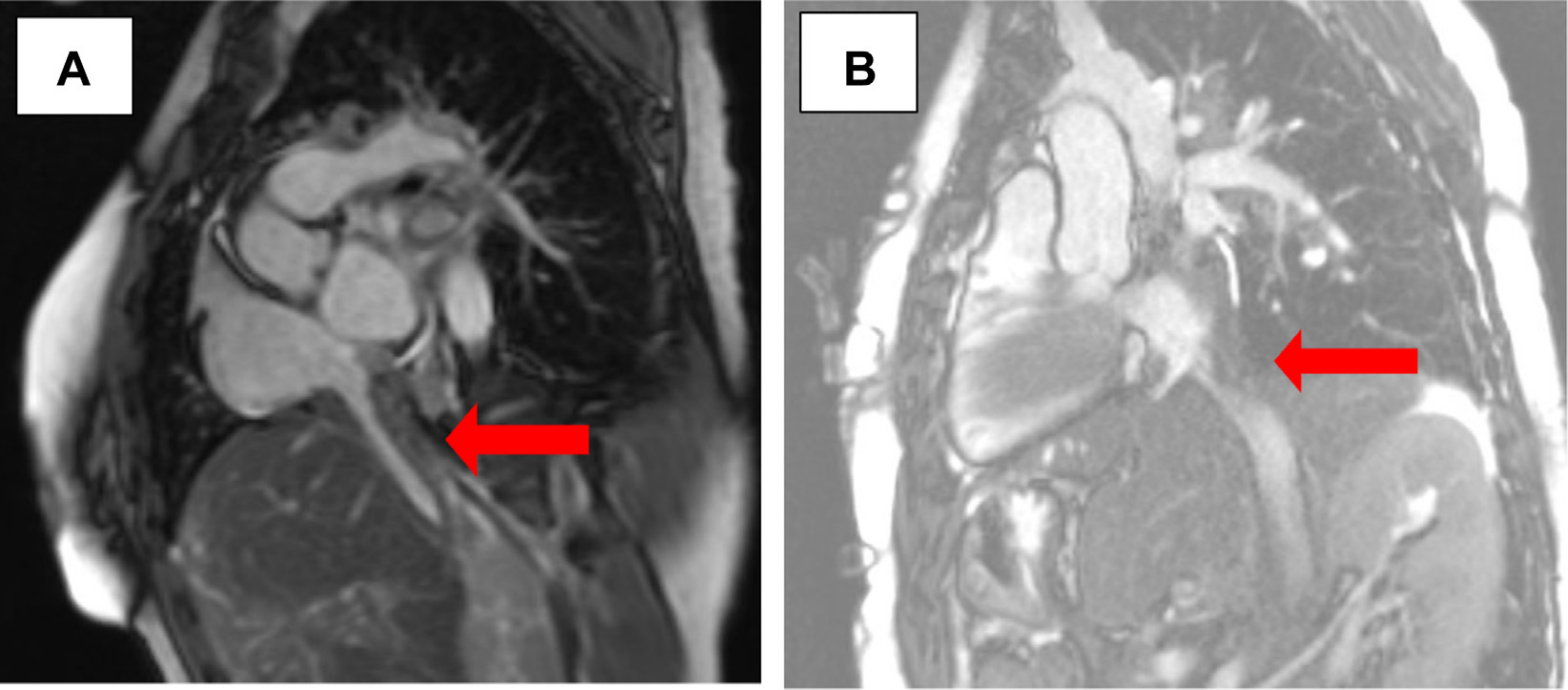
Cardiac Magnetic Resonance Imaging Eccentric wall thickening of the posterior RA with extension into the IVC, where there is circumferential wall thickening and subsequent luminal narrowing. These findings are enhanced with gadolinium. Abbreviations as in Figure 1.
